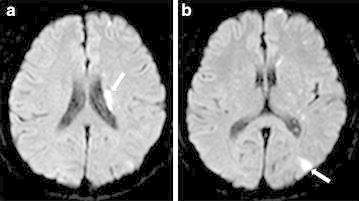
Brain diffusion-weighted magnetic resonance imaging. a Multiple embolic infarction in left basal ganglia are seen (*arrow*). b In parietotemporal lobe (*arrow*)
